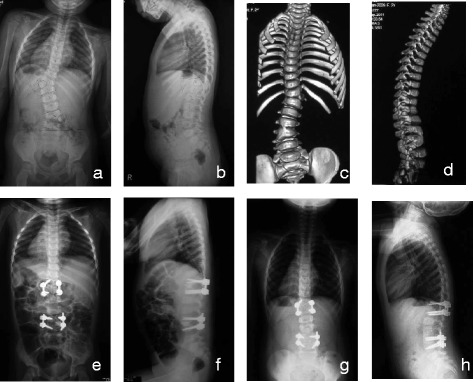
An infant with congenital scoliosis. Preoperative radiographs (a, b) and CT 3D reconstruction (c, d) showed fully segmented hemivertebra of T12/L1 and semi-segmented hemivertebra of L4/L5 with coronal segmental scoliosis of 40° and 32° and segmental kyphosis of 32°. Post-operational radiographs (e, f) showed T12/L1 and L4/L5 hemivertebra resection and internal fixation, and the scoliosis decreased to 5° and 2°, and the kyphosis decreased to 11°. Radiographs at 4-year follow-up (g, h) showed good spinal balance with coronal segmental scoliosis of 9° and 2° and segmental kyphosis of 5°
